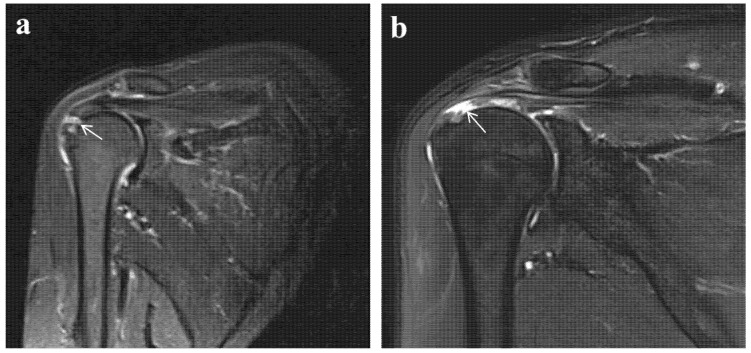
Representative MRI of traumatic and degenerative rotator cuff tear. (a) Oblique sagittal T2-weighted image demonstrating marked bone marrow edema within the greater tuberosity, visualized as a broad area of high signal intensity. The tear gap is filled with hyperintense fluid, and the torn tendon edge maintains a relatively preserved tension rather than appearing diffusely frayed or retracted, further supporting an acute injury pattern. (b) Coronal T2-weighted fat-suppressed image demonstrates a full-thickness tear of the supraspinatus tendon with significant retraction. Note the frayed, thinned, and irregular morphology of the tendon stump, characteristic of chronic degenerative changes. Note: Arrows indicate the site of the tear.
